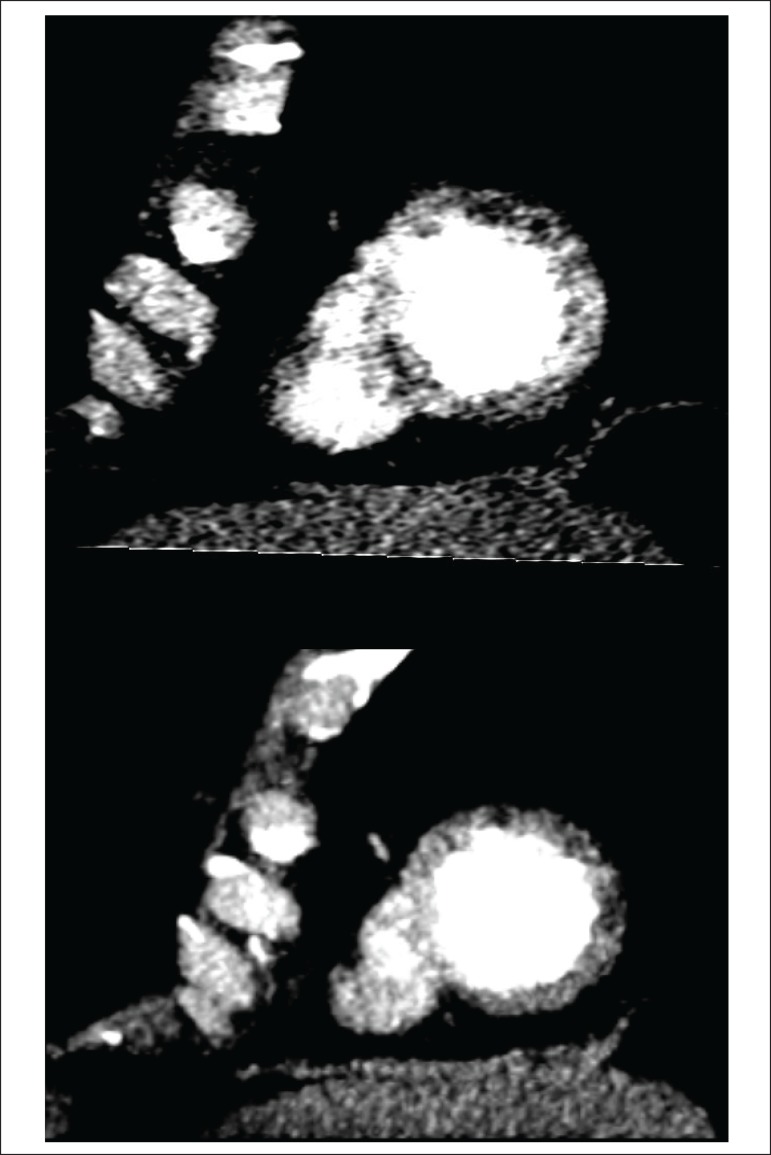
64-channel Cardiac Computed Tomography, effort and rest stage, reveals
